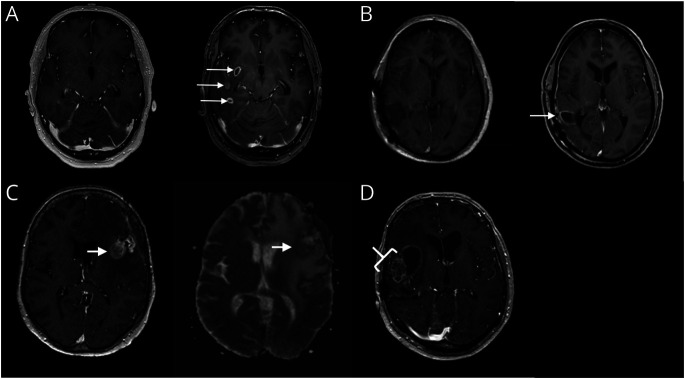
MRI Characteristics With Significant Predictive Value (A) A 64-year old man with an isocitrate dehydrogenase (IDH)–wild-type (WT) glioblastoma treated with radiotherapy. Left: Preoperative baseline MRI. Right: Follow-up MRI (93 days after baseline) with multiple new contrast enhancements. (B) A 58-year-old man with an IDH-WT glioblastoma treated with temozolomide-based chemoradiation. Left: Baseline MRI of the surgical cavity after resection. Right: Follow-up MRI (91 days after baseline) with increased marginal enhancement of the surgical cavity. (C) A 65-year old woman with a glioblastoma, IDH status unknown, treated with radiotherapy. Left: T1-MRI with contrast agent. Right: Isointense ADC signal compared with healthy white matter. (D) A 66-year old man with an IDH-mutated astrocytoma grade 4 treated with temozolomide-based chemoradiation: soap bubble enhancement (small regions of necrosis^17^).
